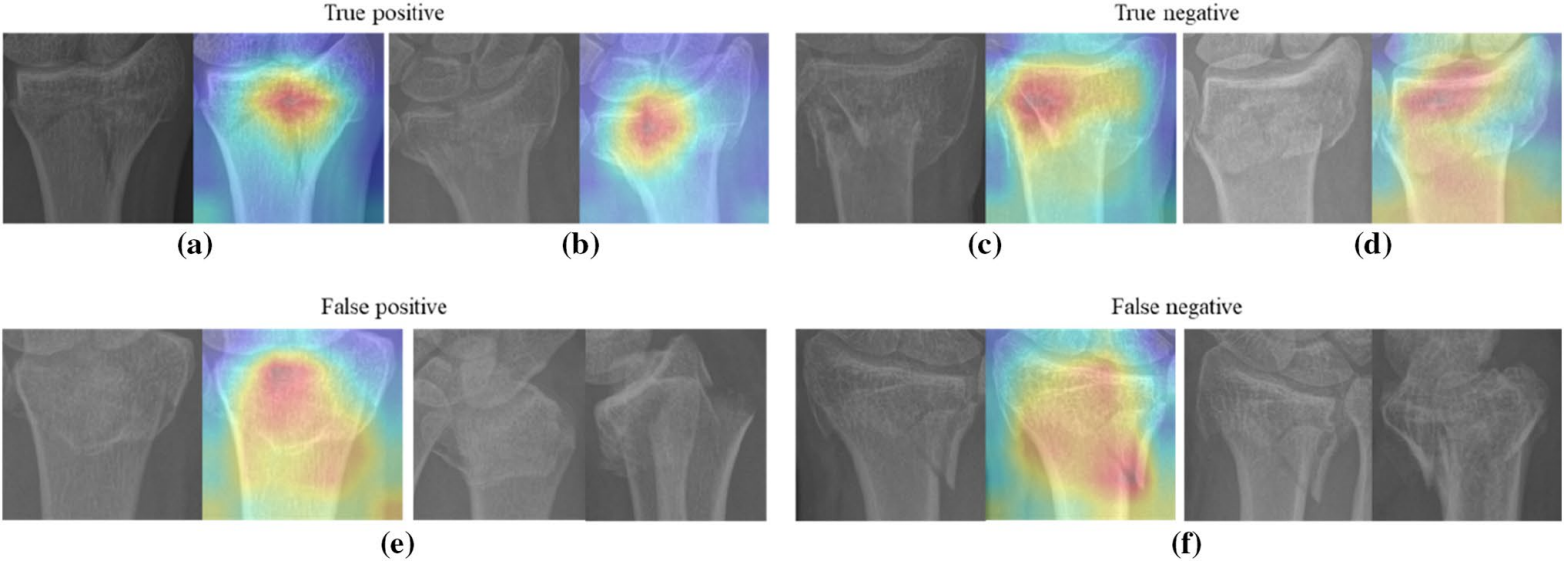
DRF classification examples with Grad-cam overlay on distal radius ROIs. a and b are true positive detections (intra-articular). c and d are true negative classifications (extra-articular). e and f are false positive and false negative classifications respectively. For the incorrectly classified examples (e) and (f), apart from the ROIs extracted from the PA view X-ray and the Grad-cam overlay (first two images for these cases), the relevant regions from the PA oblique and lateral views are also shown on the right side to provide additional information
